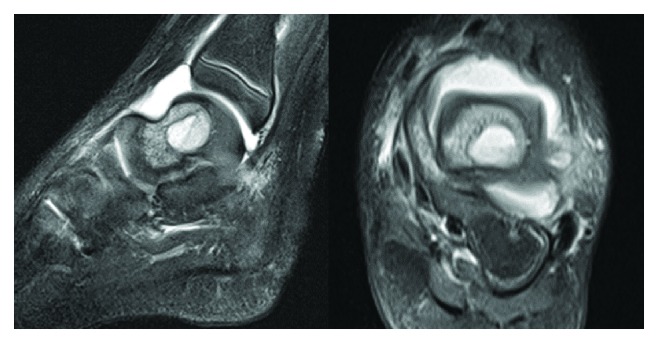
MRI T2-weighted lateral and axial cuts of the ankle.
